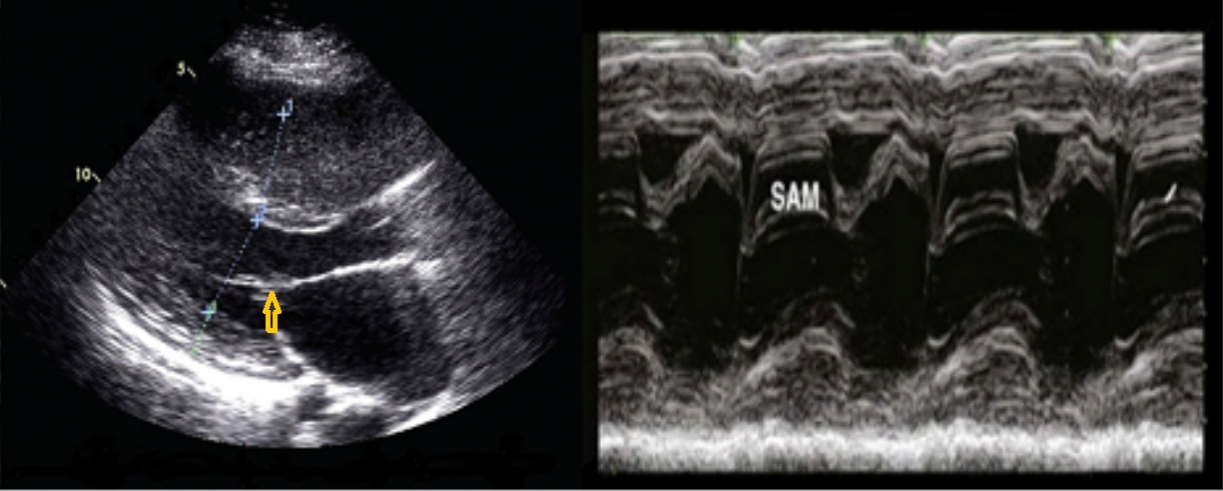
2-D Echocardiography Parasternal long-axis view of the patient’s left ventricle showing asymmetric interventricular septum hypertrophy *(left)*, and M-mode showing the systolic anterior motion (SAM) of the mitral valve leaflet *(right)*
